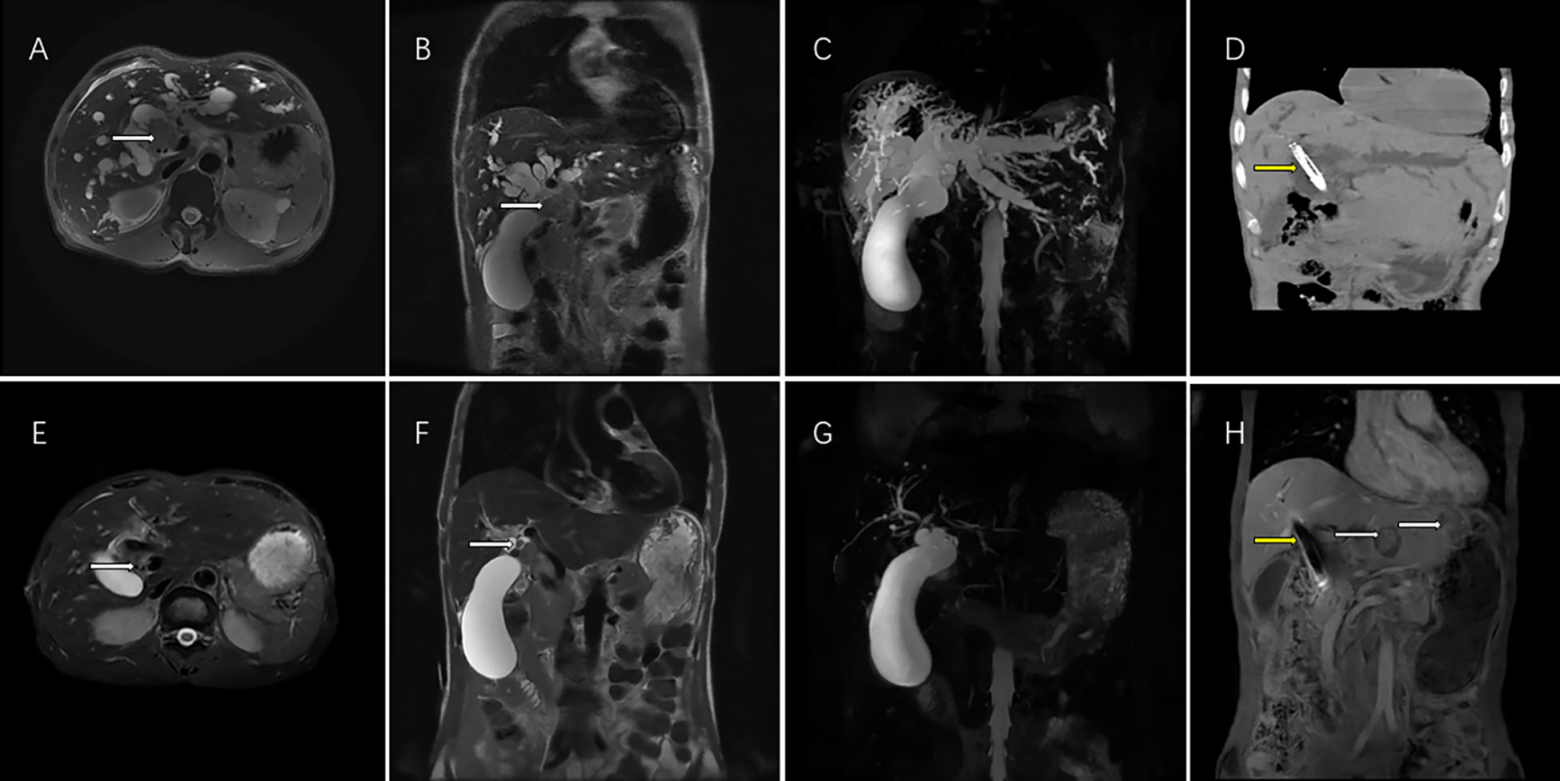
This magnetic resonance (MR) images showed the imaging feature of 62-year-old men with perihilar cholangiocarcinoma accompanied by malignant obstructive jaundice. T2 weighted cross sectional imaging (A) and T2 weighted coronal imaging (B) showed the bile duct tumor of the hilar region (white arrow) with bile duct dilatation before interventional treatment plus lenvatinib with PD-1inbhibitor therapy; Magnetic resonance cholangial pancreatography (MRCP) showed thick intrahepatic dilated bile ducts and a large gallbladder (C); Computed tomography showed biliary stenting with iodine-125 seed strand (yellow arrow) were in good position 1 month after the operation (D). T2 weighted cross sectional imaging (E), T2 weighted coronal imaging (F) showed that the bile duct tumor volumes of the hilar region shrank (white arrow), the bile duct returned to normal size and achieved PR according to the mRECIST criteria after 5 courses of interventional treatment plus lenvatinib plus PD-1inbhibitor therapy. MRCP showed the normalization of the intrahepatic bile ducts after treatment (G). Although hepatic portal tumor was well controlled (yellow arrow), two new intrahepatic metastatic lesions appeared (yellow arrow) in the left liver 12 months after interventional treatment plus lenvatinib with PD-1inhibitor therapy and achieved PD according to the mRECIST criteria (H). PR, partial response; mRECIST, the Modified Response Evaluation Criteria in Solid Tumors. PD, progression disease; mRECIST, the Modified Response Evaluation Criteria in Solid Tumors; PD-1, programmed death-1.
